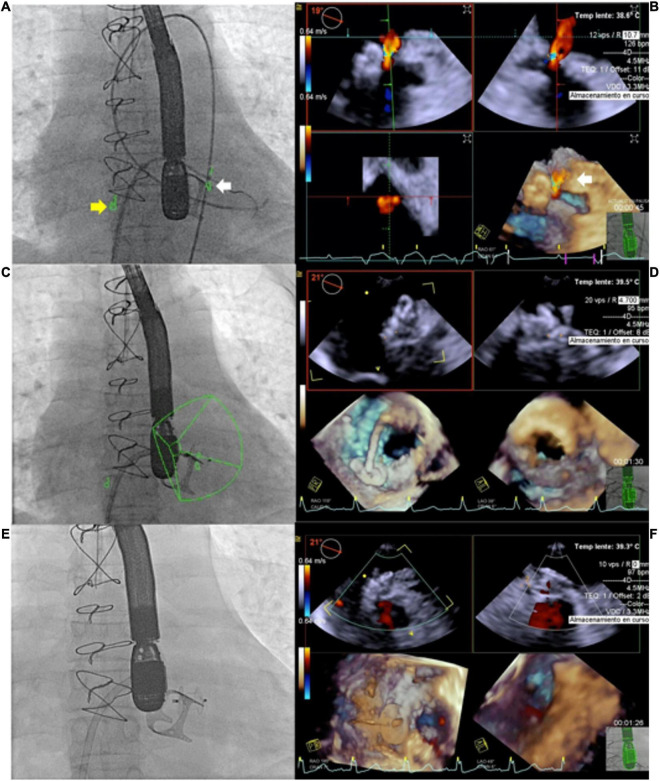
Use of echocardiography-fluroroscopy fusion (EFF) to facilitate mitral paravalvular leak (PVL) closure. (A,B) Anatomical markers placed on fluoroscopy and echocardiography. Marker 1 (yellow arrow) indicate position of transeptal puncture. Marker 2 (white arrow) indicate site of mitral PVL. (B) Color flow Doppler confirming mitral PVL. Combined EFF image allows efficient crossing of PVL (C,D). Continuous feedback from EFF facilitates alignment of delivery sheath and device deployment. (E) Fluoroscopy showed good device position post release, (F) confirmed by echocardiography and no residual leak was observed. *Courtesy of Dr. Juan Pablo Sandoval, Instituto Nacional de Cardiologia Ignacio Chavez, Mexico City, Mexico*.
